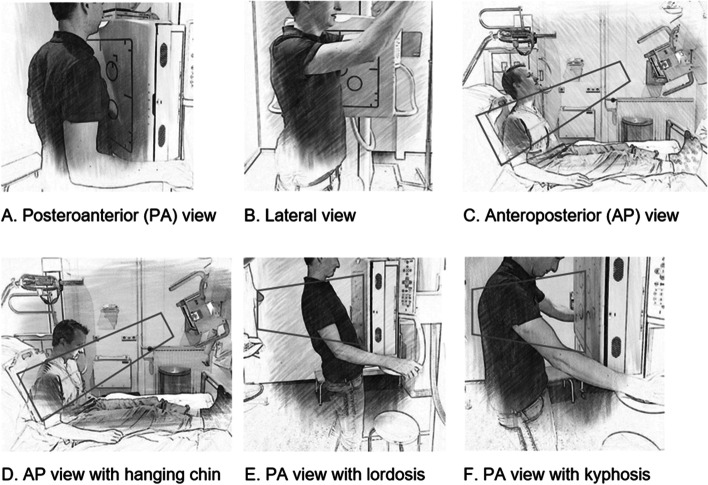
Projection views of chest X-ray (Figure by author). A and B show the standard projections of the chest X-ray. The patient is standing against the detector. C shows an AP projection without the presence of a hanging chin sign. D, E and F show examples in which a hanging chin sign could be seen on the chest X-ray
